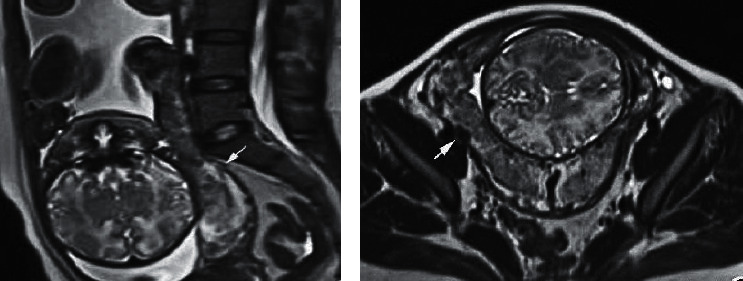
The MRI image of a partial placenta previa case. (a) The sagittal plane. (b) The transverse plane.
